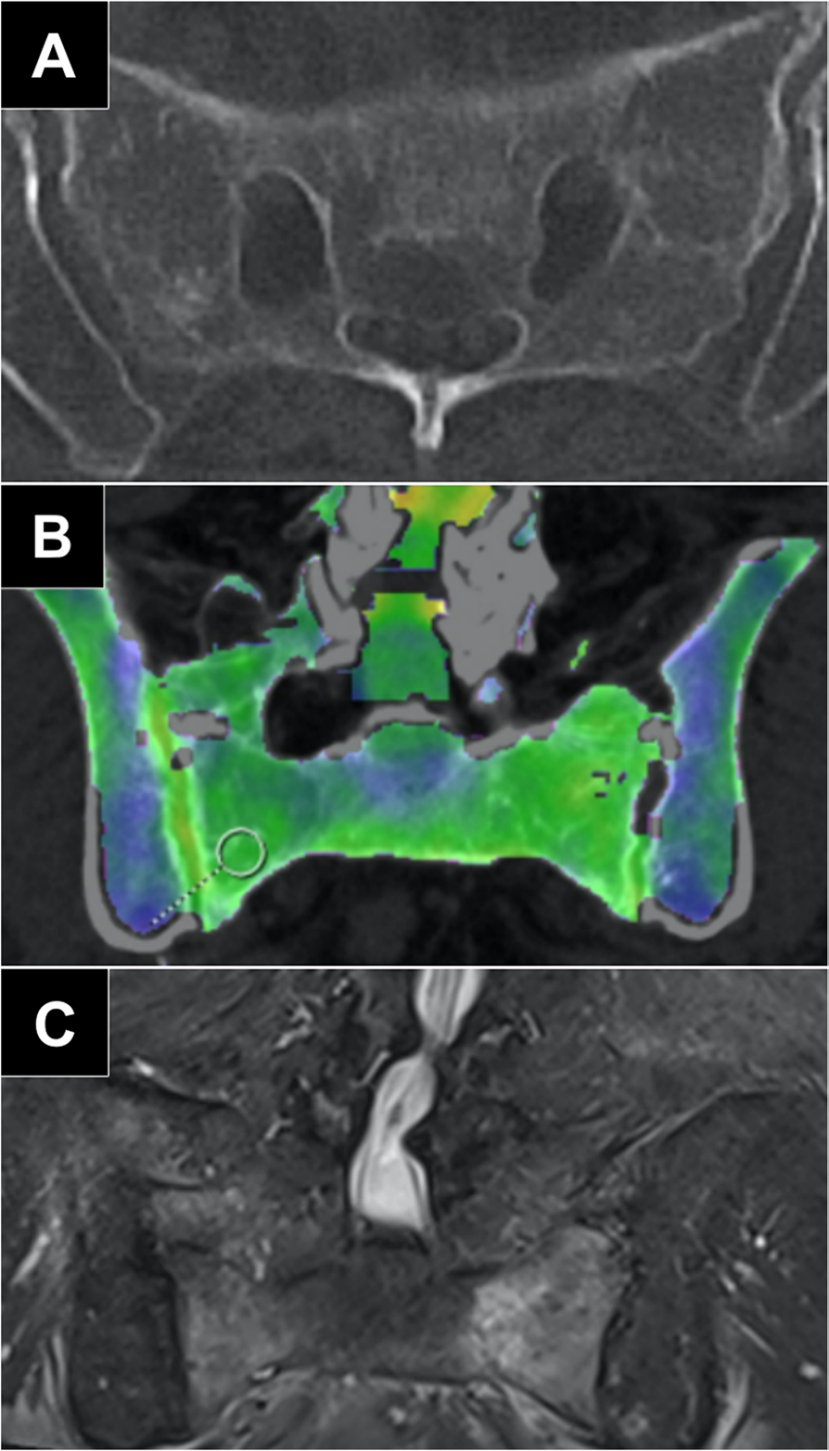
a Axial CT image that fails to demonstrate a discrete fracture line in the sacrum. b Color-coded coronal DECT reconstruction image with region-of-interest measurement in the right inferior region of the sacrum. Blue represents lower HU values of approximately -50 HU, whereas yellow/light green indicates higher HU values around + 10 HU. c Coronal T2-weighted STIR sequence demonstrates edema in the same locations
